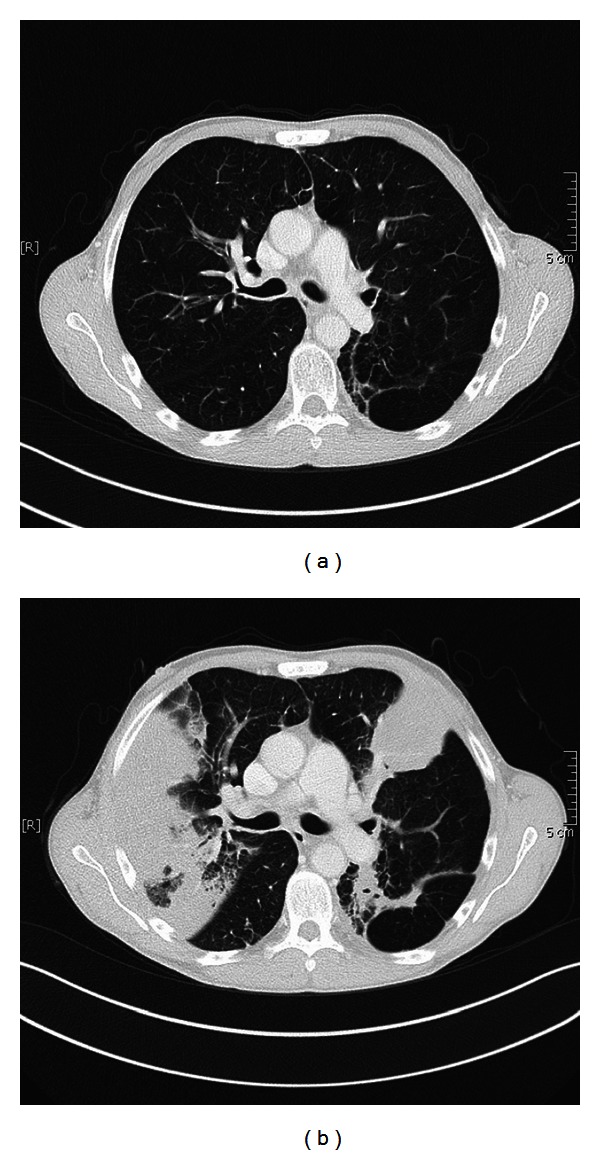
(a). CT scan 4 weeks before the intervention of endoscopic lung volume reduction revealed severe heterogeneous centrilobular lung emphysema with bilateral involvement of the upper lobes. (b) CT scan 3 days after the intervention of AeriSeal revealed the induced atelectasis of the bilateral upper lobes.
